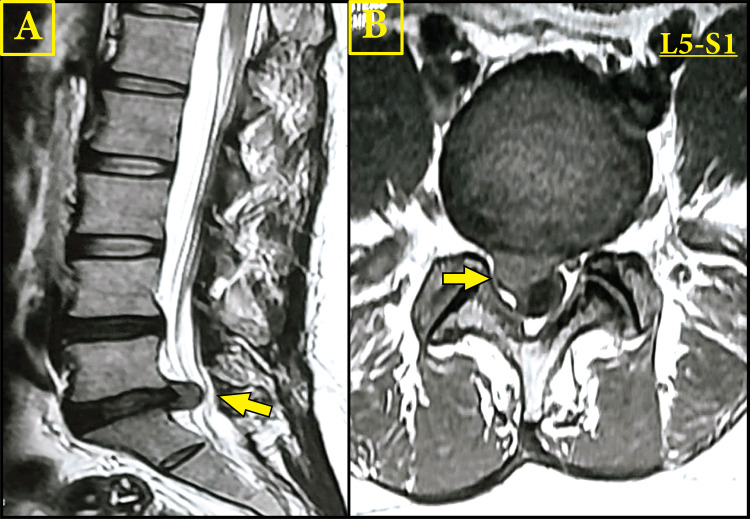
An MRI scan of the lumbar spine Magnetic resonance imaging demonstrated an L5-S1 moderate-sized right-sided subarticular disc extrusion compressing the traversing nerve roots on the right side of the spinal canal. A: T2 sagittal MRI shows the lumbar spine with an arrow indicating a disc herniation; B: T1 axial (cross-sectional) MRI view of the L5-S1 level, with the arrow pointing to the corresponding herniated disc material.
